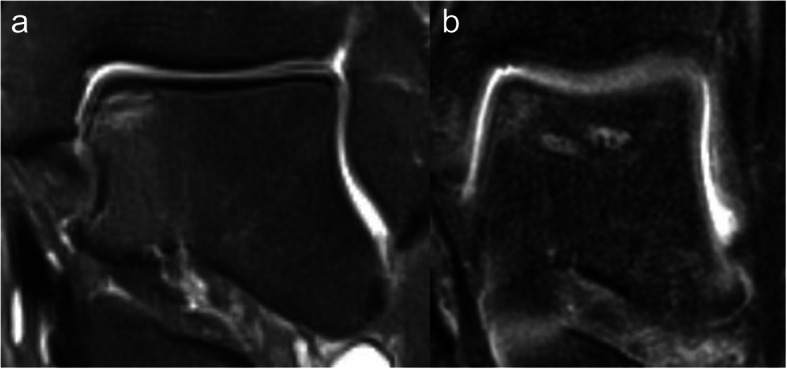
a/b MRI 6 months postoperatively after standard procedure with visual drilling channels and 2 years postoperatively with good bone union
